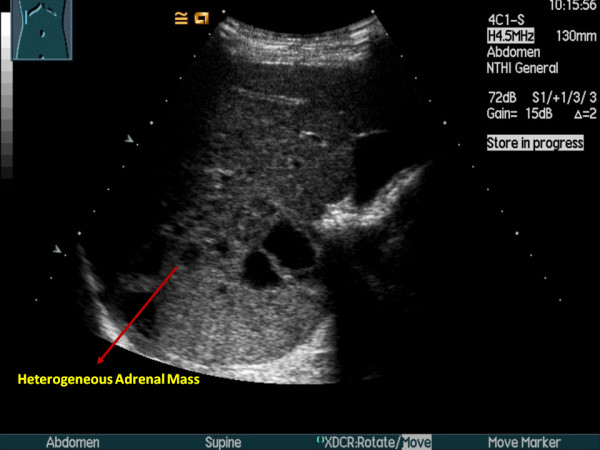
Abdominal ultrasound depicting a large mixed cystic/solid right adrenal mass
